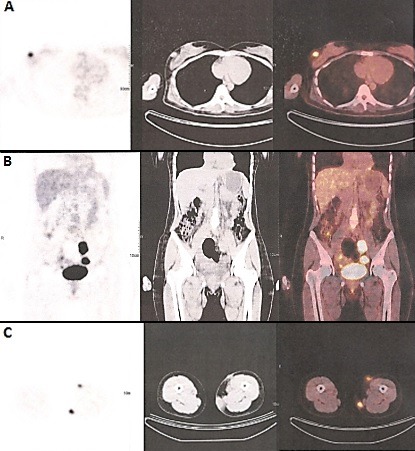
Bilan d'extension par TEP-TDM: (A) Nodule sous cutané du sein droit hypermétabolique (SUVmax à 6,55); (B) adénopathies hypermétaboliques iliaques internes bilatérales (SUVmax: à droite 9,29 et à gauche 22,31); (C) nodules cutanés hypermétaboliques de la face antérointerne et interne de la cuisse gauche (SUVmax respectif à 4,23 et 8,84)
